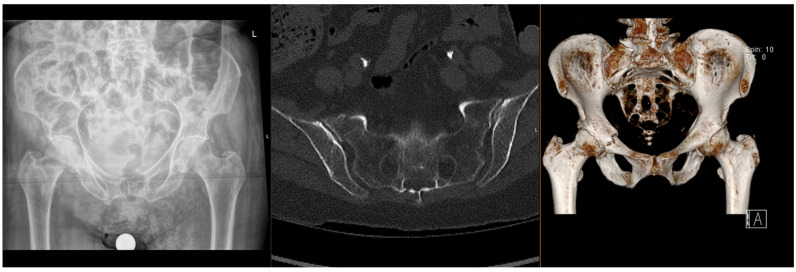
Radiographs and CT images demonstrating common FFP: lateral compression type.
